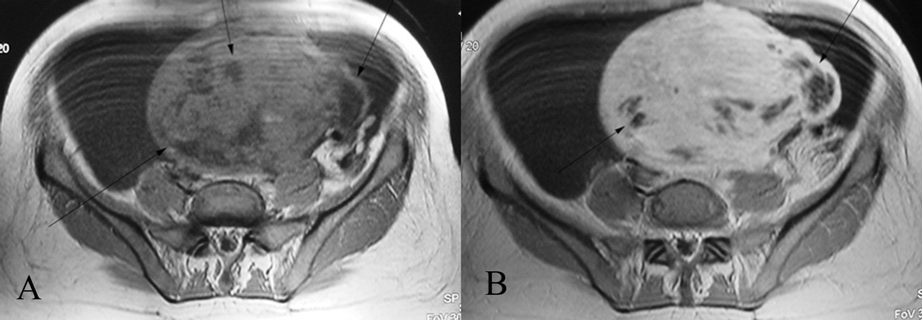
(A) Axial T1-weighted spin echo and (B) contrast-enhanced axial T1-weighted spin echo magnetic resonance image which show similar enhancement of the normal myometrium and mass. The foci of high signal intensity on the T2-weighted image show no enhancement on the contrast-enhanced images representing areas of cystic degeneration (arrows).
